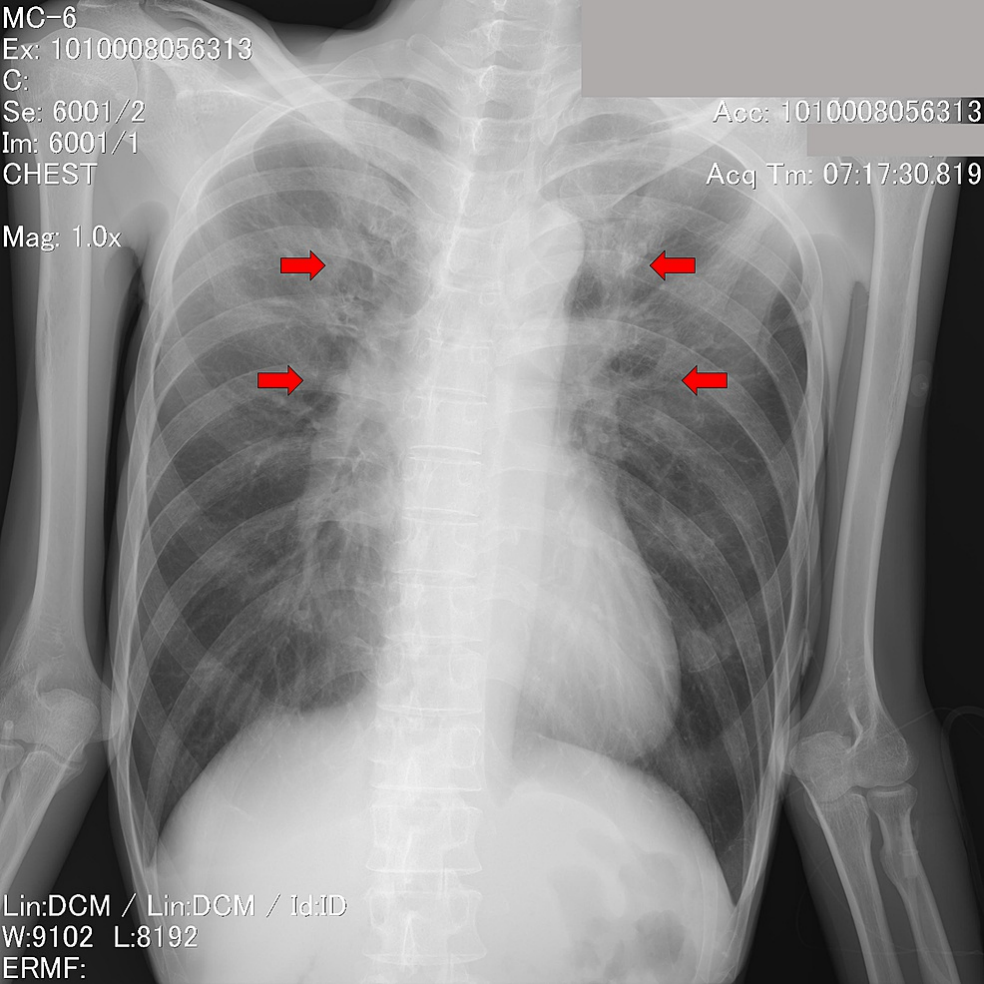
Chest X-ray at admission Initial chest X-ray demonstrated mild interstitial pulmonary edema (red arrows).
